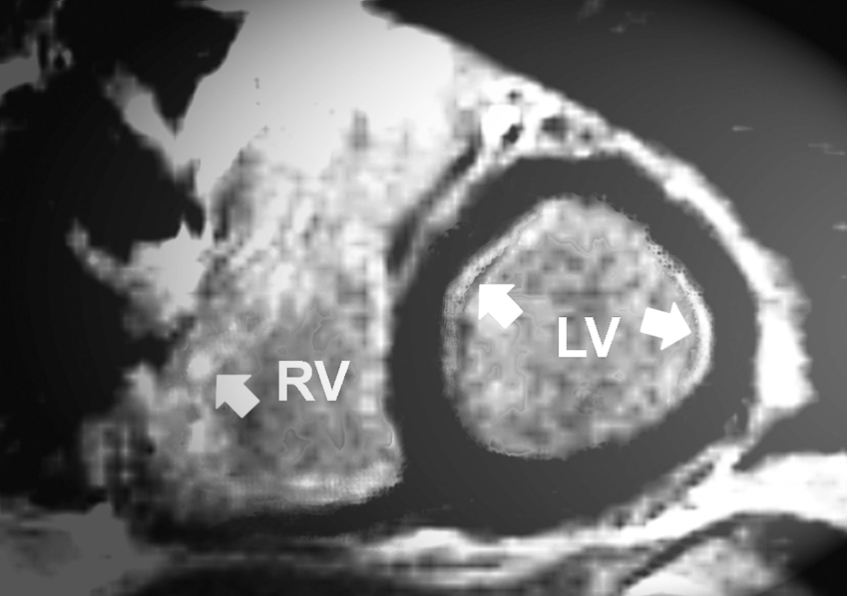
Magnetic resonance imaging of patient number 6 (short axis) showing a signal enhancement of the septal (arrow) and slightly of the basolateral region (arrow) of the left ventricular myocardium (late enhancement, segmental inversion recovery - TurboFLASH 2D image). RV: right ventricle. LV: left ventricle.
